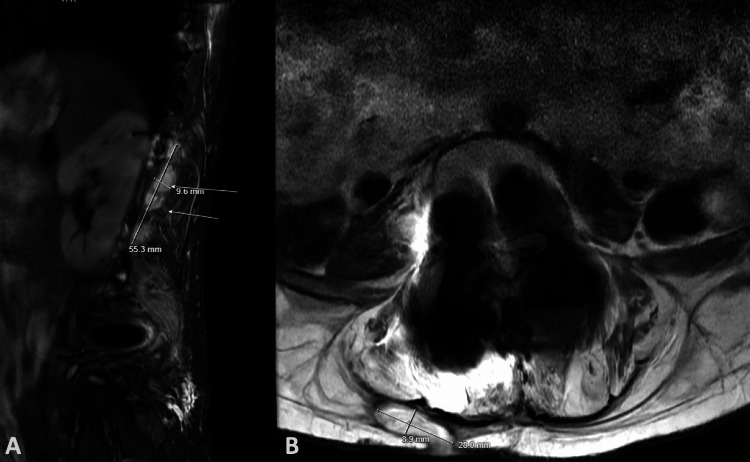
(A) Sagittal T2-weighted MRI showing a left-sided T11-L2 multilocular fluid collection lateral to the hardware and (B) axial cut of the same study showing a right-sided paraspinal muscle focal fluid collection spanning the L3-5 levels with a draining sinus.
